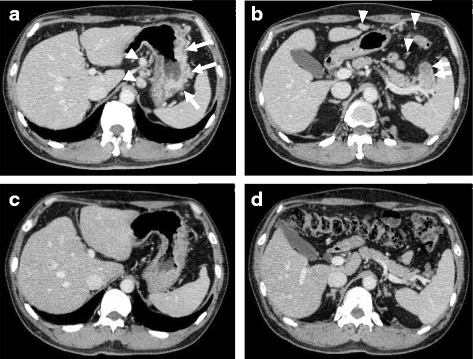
Computed tomography findings. a Thickening of the gastric wall, high density of fat around the gastric wall (*arrow*), and perigastric lymphadenopathy (*arrowhead*). b Perigastric lymphadenopathy (*arrow*) and multiple disseminated lesions (*arrowhead*). c Improvement of gastric wall thickening after chemotherapy. d Shrinkage of lymphadenopathy and disappearance of disseminated lesions after chemotherapy
